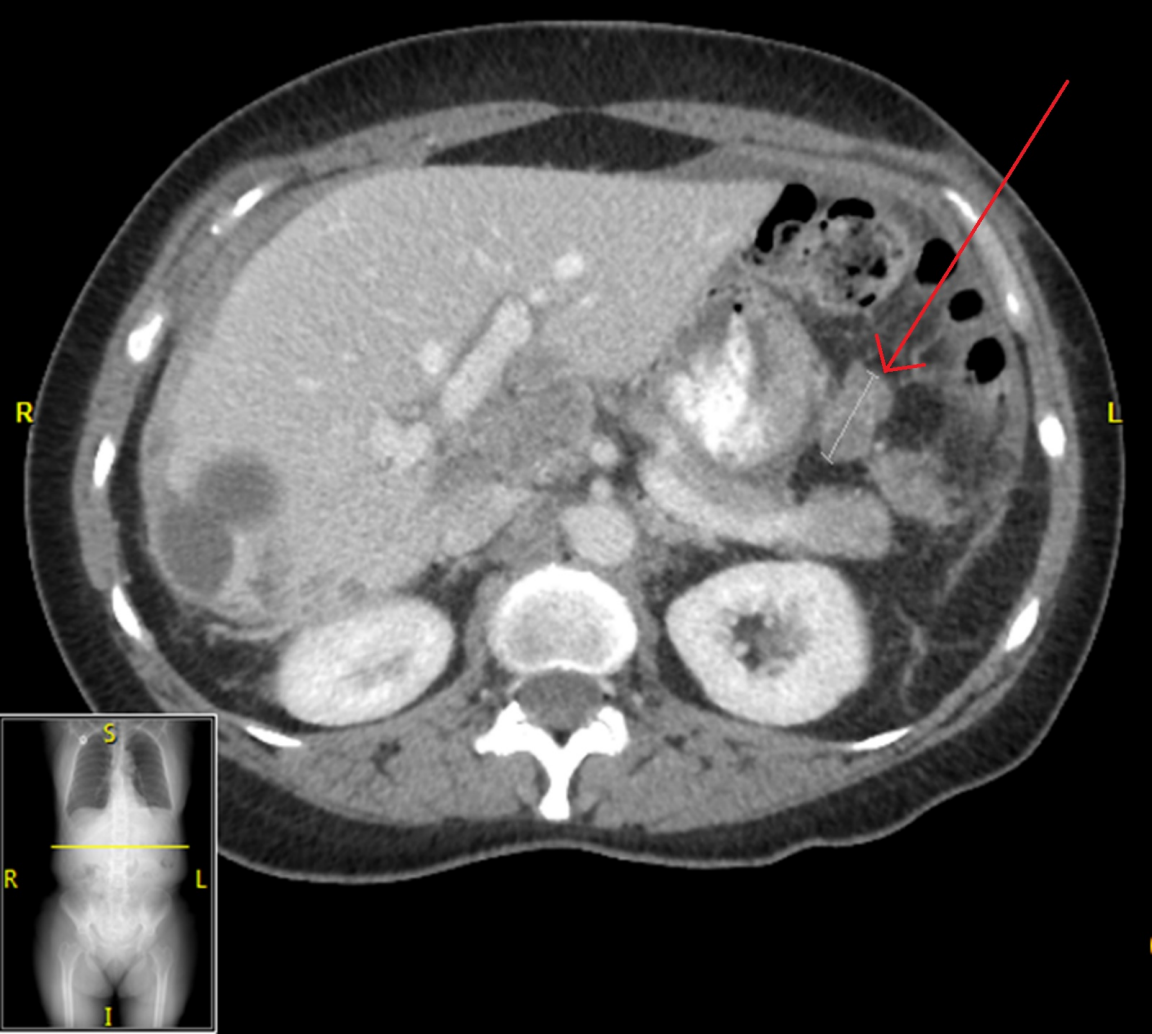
Abdominal CT of Patient 2 prior to starting evofosfamide, demonstrating target lesion of 2.7 cm in maximal dimension; CA-125 10,000 U/ml.
